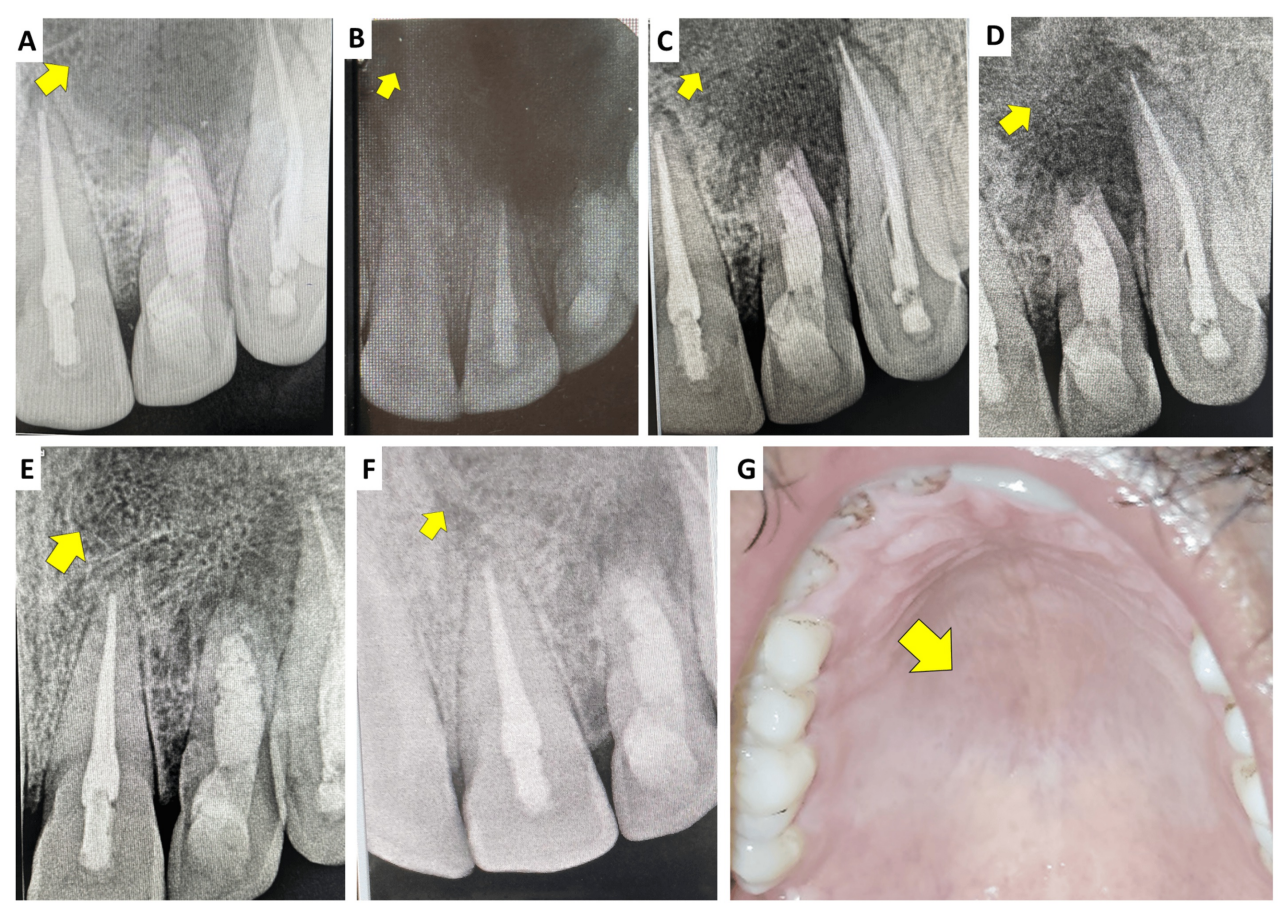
(A) Immediate postoperative radiograph after obturation with Biodentine and follow-up radiographs after (B) three months, (C) six months, (D) 12 months, (E) 18 months, and (F) 24 months and (G) clinically healed lesion at 24 months
